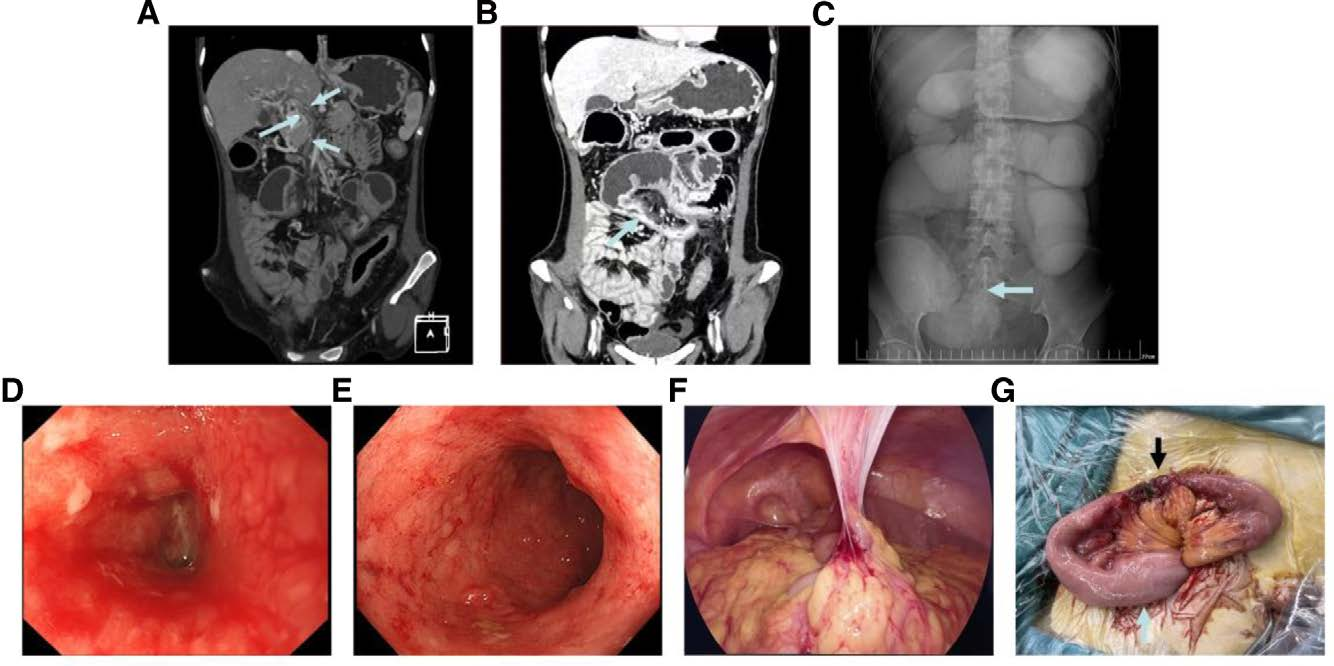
(A) Portal and superior mesenteric vein thrombosis (blue arrow). (B) A long segment of intestinal stenosis (blue arrow) with significant proximal bowel dilatation. (C) Digestive tract radiography revealed localized small bowel stenosis with a bird-beak sign (blue arrow). (D) Enteroscopy revealed circumferential jejunal stenosis with ulceration and obstruction. (E) Mucosal congestion and edema of the whole colon, loss of vascular texture and normal structure of the colon. (F) A band of adhesions at a narrow site of the small intestine. (G) A 10 cm jejunal stenosis (black arrow) with significant proximal intestinal dilation and edema (blue arrow).
